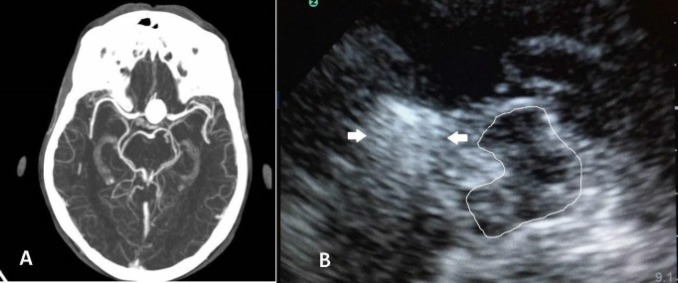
A. Axial brain computed tomography (CT)-angiography showing a giant left (Lt) distal internal carotid artery (ICA) aneurysm. B. Axial transcranial sonogram (mesencephalic level): Butterfly-shaped midbrain was encircled for better visualization (solid lines). Arrows indicate the coiled distal ICA aneurysm.
